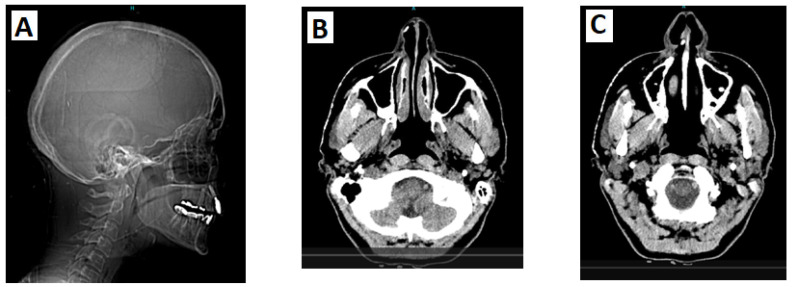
(A) CT scan of the head and neck (sagittal plane) reveals a bony projection near the anterior border of the right maxillary sinus, whereas the inferior turbinate shows slight calcification/thickening; (B) CT brain angiography shows a focal calcification in the lateral cartilaginous wall of the right inferior nasal cavity; (C) CT brain angiography reveals an osteophytic spur at the anterior border of the right maxillary sinus that projects into the right nasal cavity.
